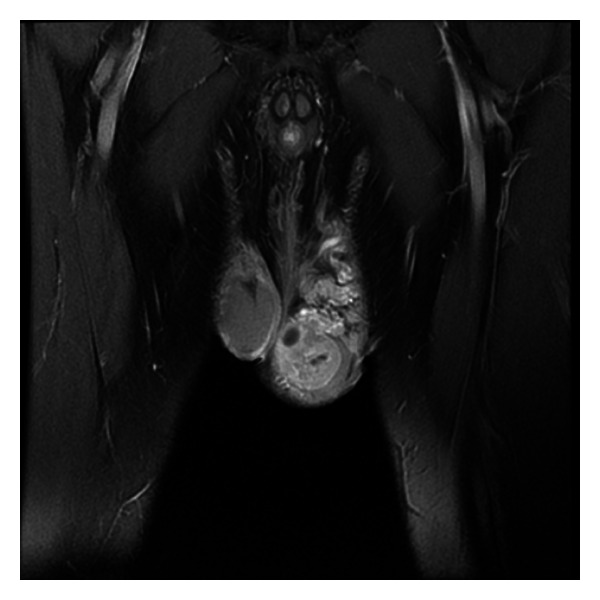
Coronal T1-weighted MRI image demonstrating a tumor that occupies the largest part of the left testis.
